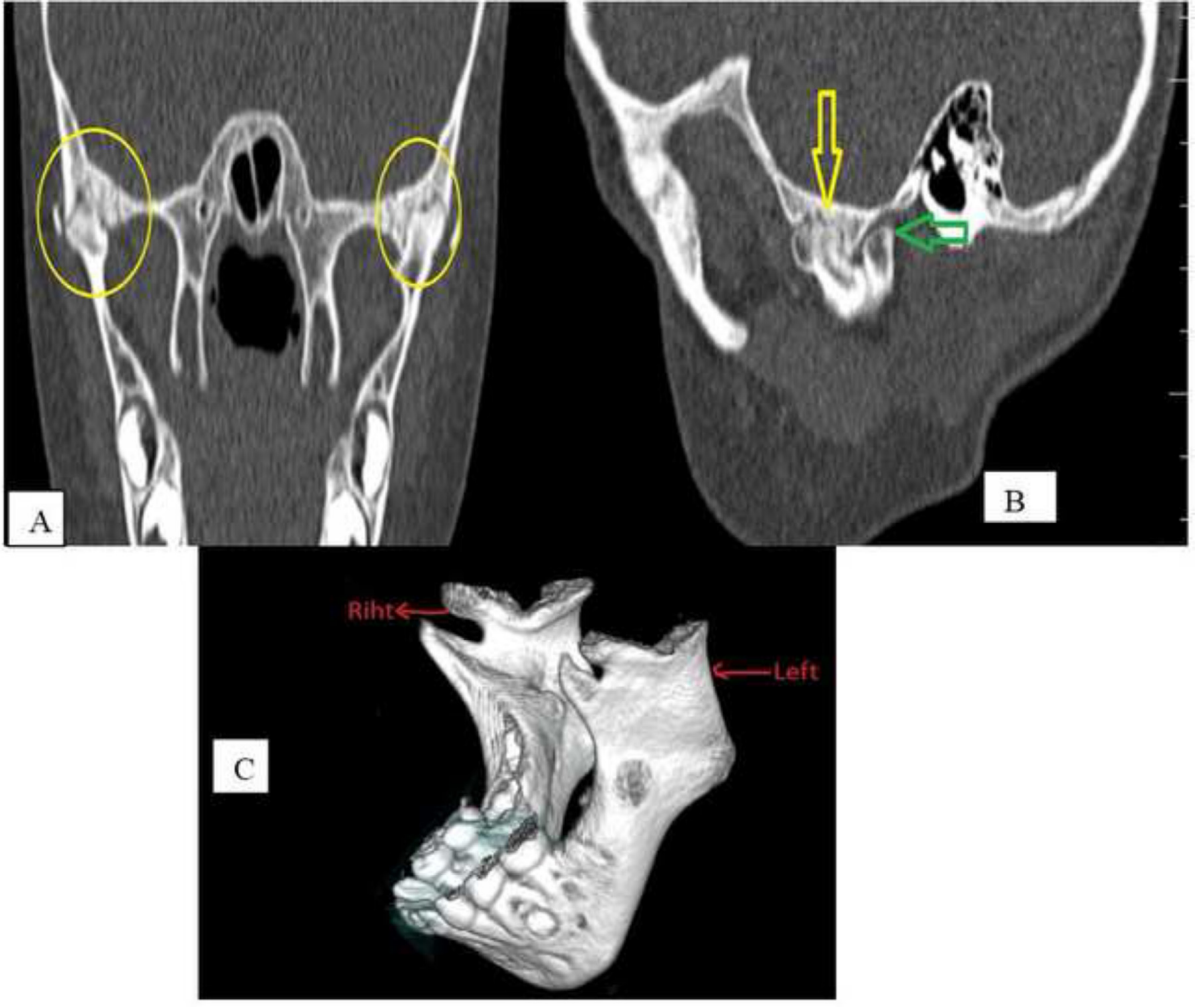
(A) Coronal reconstructed CT section of bilateral TMJ. Bilateral anterior head shows fibrous ankylosis with the temporal bone (yellow circles). (B) Sagittal reconstructed CT section through the lateral aspect of the right TMJ. The posteriorly placed condylar head is articulating with the temporal fossa (green arrow). The anterior head shows fibrous ankylosis with the temporal bone (yellow arrow). (C) Bilateral bifid mandibular condyle in 3-dimensional reconstructed image.
